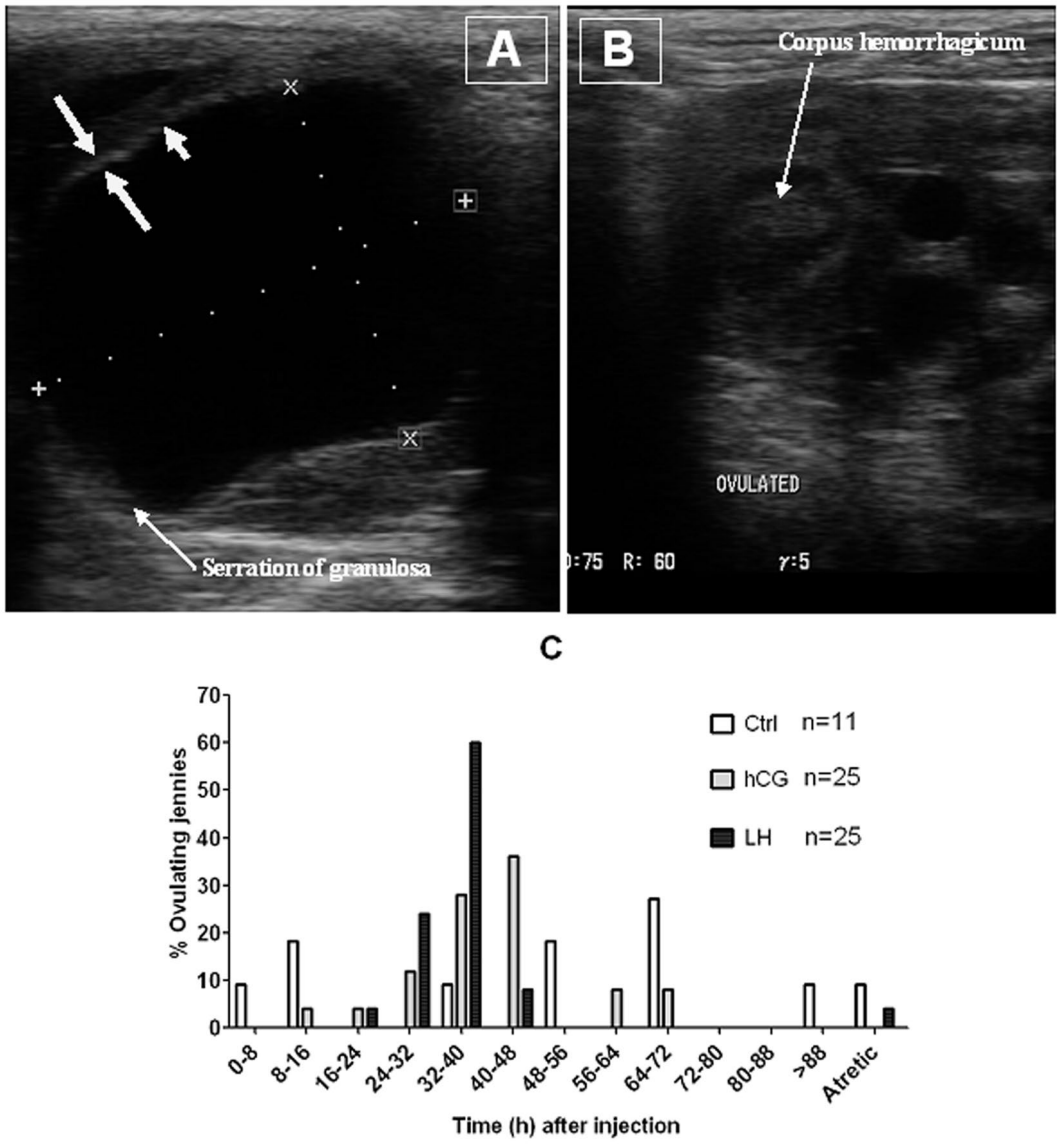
Distribution of ovulations following the ovulation induction treatment of jennies. When the dominant follicle diameter on an ovary reached 35 mm, jennies in control (Ctrl, n = 11), hCG (n = 25) and LH (n = 25) groups were injected intramuscularly with 5 ml saline alone or saline containing 3000 IU hCG or 400 IU LH, respectively. Then, follicle development was checked by ultrasonic imaging every 8 h until the dominant follicle ovulated. (A) Ultrasonographic image of a large preovulatory follicle with a thick echogenic border. As ovulation approached, the granulosa layer (large arrows) became thicker (more echogenic), and segments of the follicular wall with an anechoic bands (short arrows) appeared. (B) Ultrasonographic image of a collapsed ovulated follicle forming a corpus hemorrhagicum after LH treatment. (C) Distribution of ovulations following different ovulation induction treatments.
